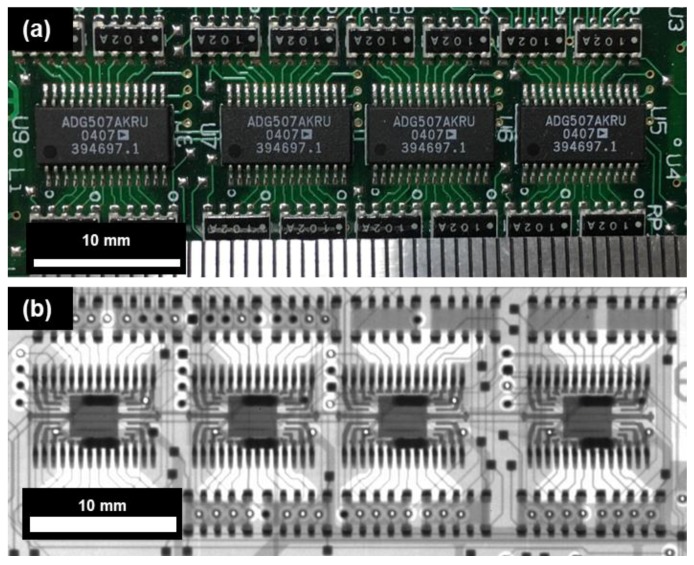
(a) The optical image and (b) X-ray image of integrated circuit taken at 55 kV/0.5 mA.
